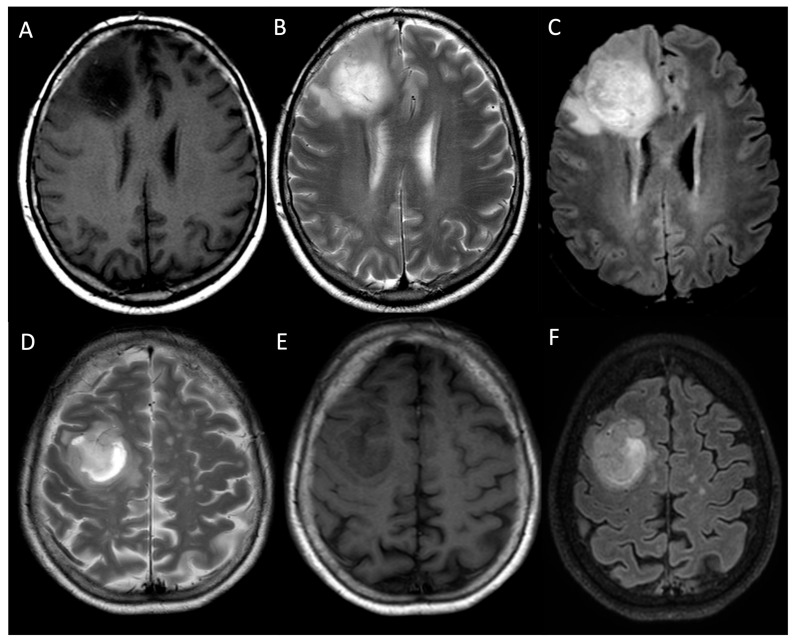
Shows a grade 4, IDH wild-type primary glioblastoma of the right frontal lobe (A–C) and a grade 4, IDH wild-type primary glioblastoma of the premotor area of the right frontal lobe (D–F). Both lesions appear inhomogeneously hyperintense on T2WI (B,D) and FLAIR (C,F), and inhomogeneously hypointense on T1WI (A,E). Peripheral vasogenic oedema appears hypointense on T1WI (A,E) and hyperintense on T2WI/FLAIR (B–D,F). *WI: weighted image; FLAIR: fluid-attenuated inversion recovery*.
